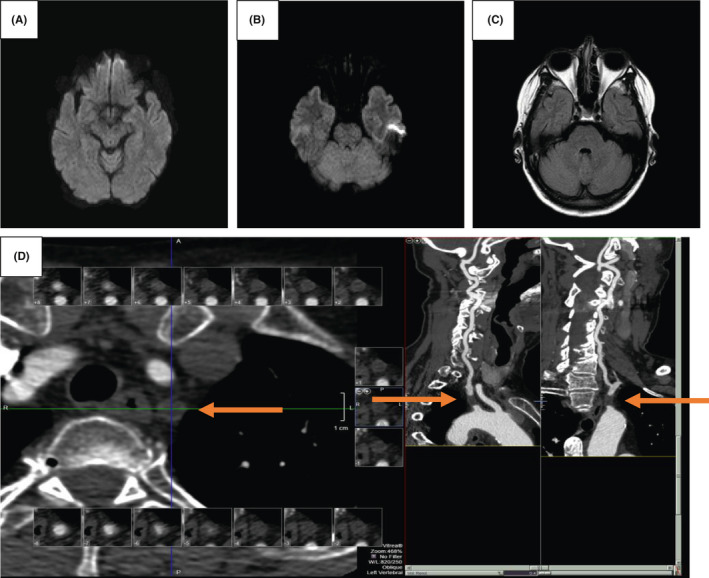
Brain MRI shows no diffusion restriction in the posterior circulation on DWI sequence (A and B), without associated T2 hyperintensity on fluid‐attenuated inversion recovery sequence (C). CTA neck shows complete occlusion of the left subclavian artery distal to its origin and proximal to the origin of the left vertebral artery (D)
